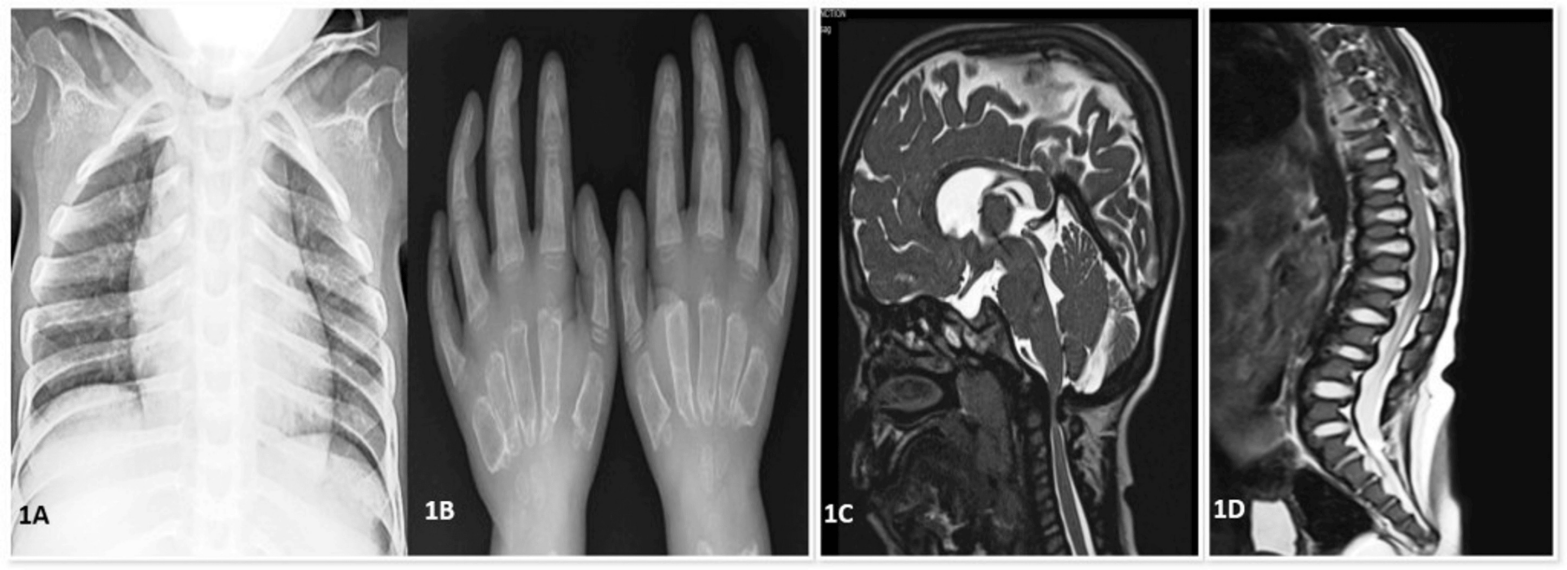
1A) Chest X-ray: Pectus excavatum deformity and short neck; 1B) Hand X-ray: diaphyseal thickening with short, wide metacarpals and dysplasia of the carpal bones, with increased scapholunar distance suggesting instability; 1C) MRI brain with cervical spine: craniosynostosis with scaphocephaly, bulging temporal squamae, small posterior fossa with the belly of the pons indented against the clivus, thickened retrodental ligaments, and reduced posterior atlantodental distance causing severe spinal canal narrowing with compressive myelopathy at C1 and the odontoid level; 1D) MRI thoracolumbar spine: vertebral bodies showing anterior beaking, prominent intervertebral disc spaces, and an increased angle between the sacrum and lumbar spine.
